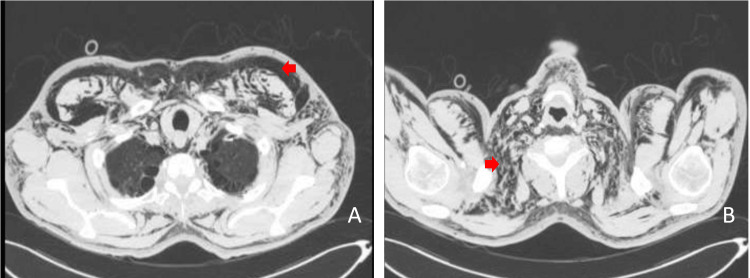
Extensive subcutaneous emphysema on chest computed tomography. Presence of air at (A) thoracic and (B) cervical levels (arrows).
